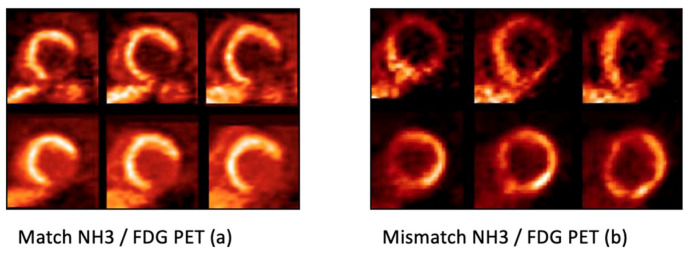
Combined perfusion (performed with N-13 ammonia) and metabolism (performed with F-18 FDG) PET study allows a differentiation between viable and non-viable myocardium. On the left side (a) is shown a perfusion/metabolism match in the posterior-lateral segments with equally reduced NH3 (top) and FDG (bottom) uptake reflecting scar in this myocardial region. On the right side (b) a perfusion/metabolism mismatch: perfusion (short axis views at the top) and metabolism (short axis views on the bottom) with reduced NH3 uptake and enhanced FDG uptake in the lateral wall. This reflects preserved viability in the hypo-perfused lateral myocardial segments.
